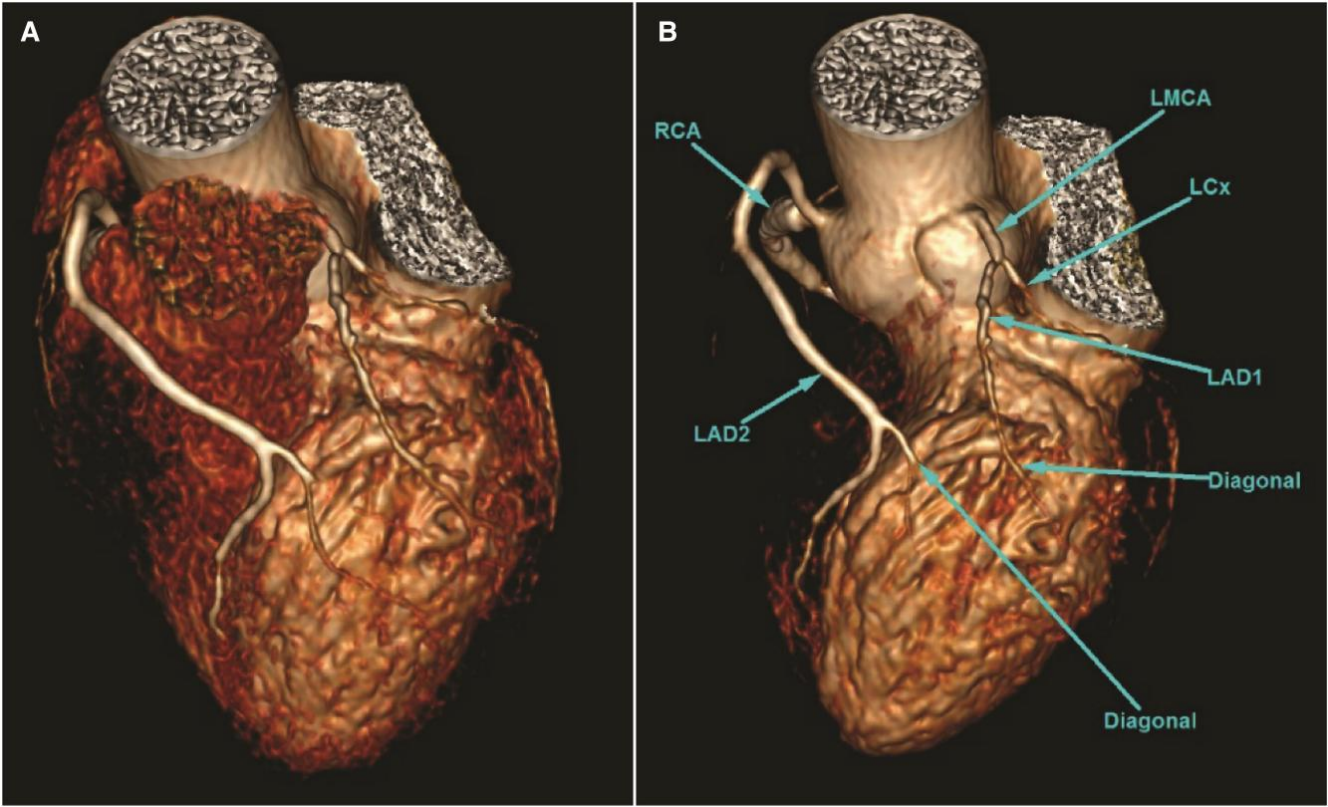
(*A*, *B*) Volume rendering computed tomography coronary image shows a type 4 dual left anterior descending artery. The short left anterior descending artery gives off the first major diagonal branch and ends in the proximal anterior interventricular groove. The aberrant long left anterior descending artery origin from the right coronary artery ostium is seen taking a pre-pulmonic course and enters the mid-anterior interventricular groove. LMCA: left main coronary artery; LAD1: short Left anterior descending artery; LAD2: Long left anterior descending artery; RCA: right coronary artery; LCX: left circumflex.
